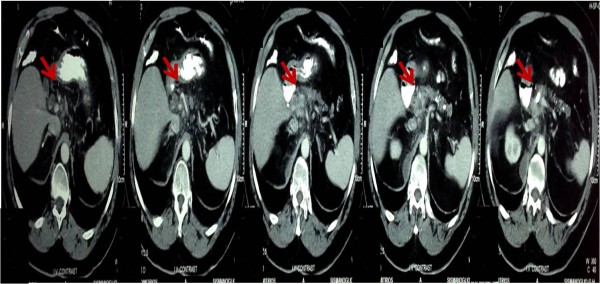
The abdominal computed tomography showed a mass at the ampulla of Vater (arrows).
